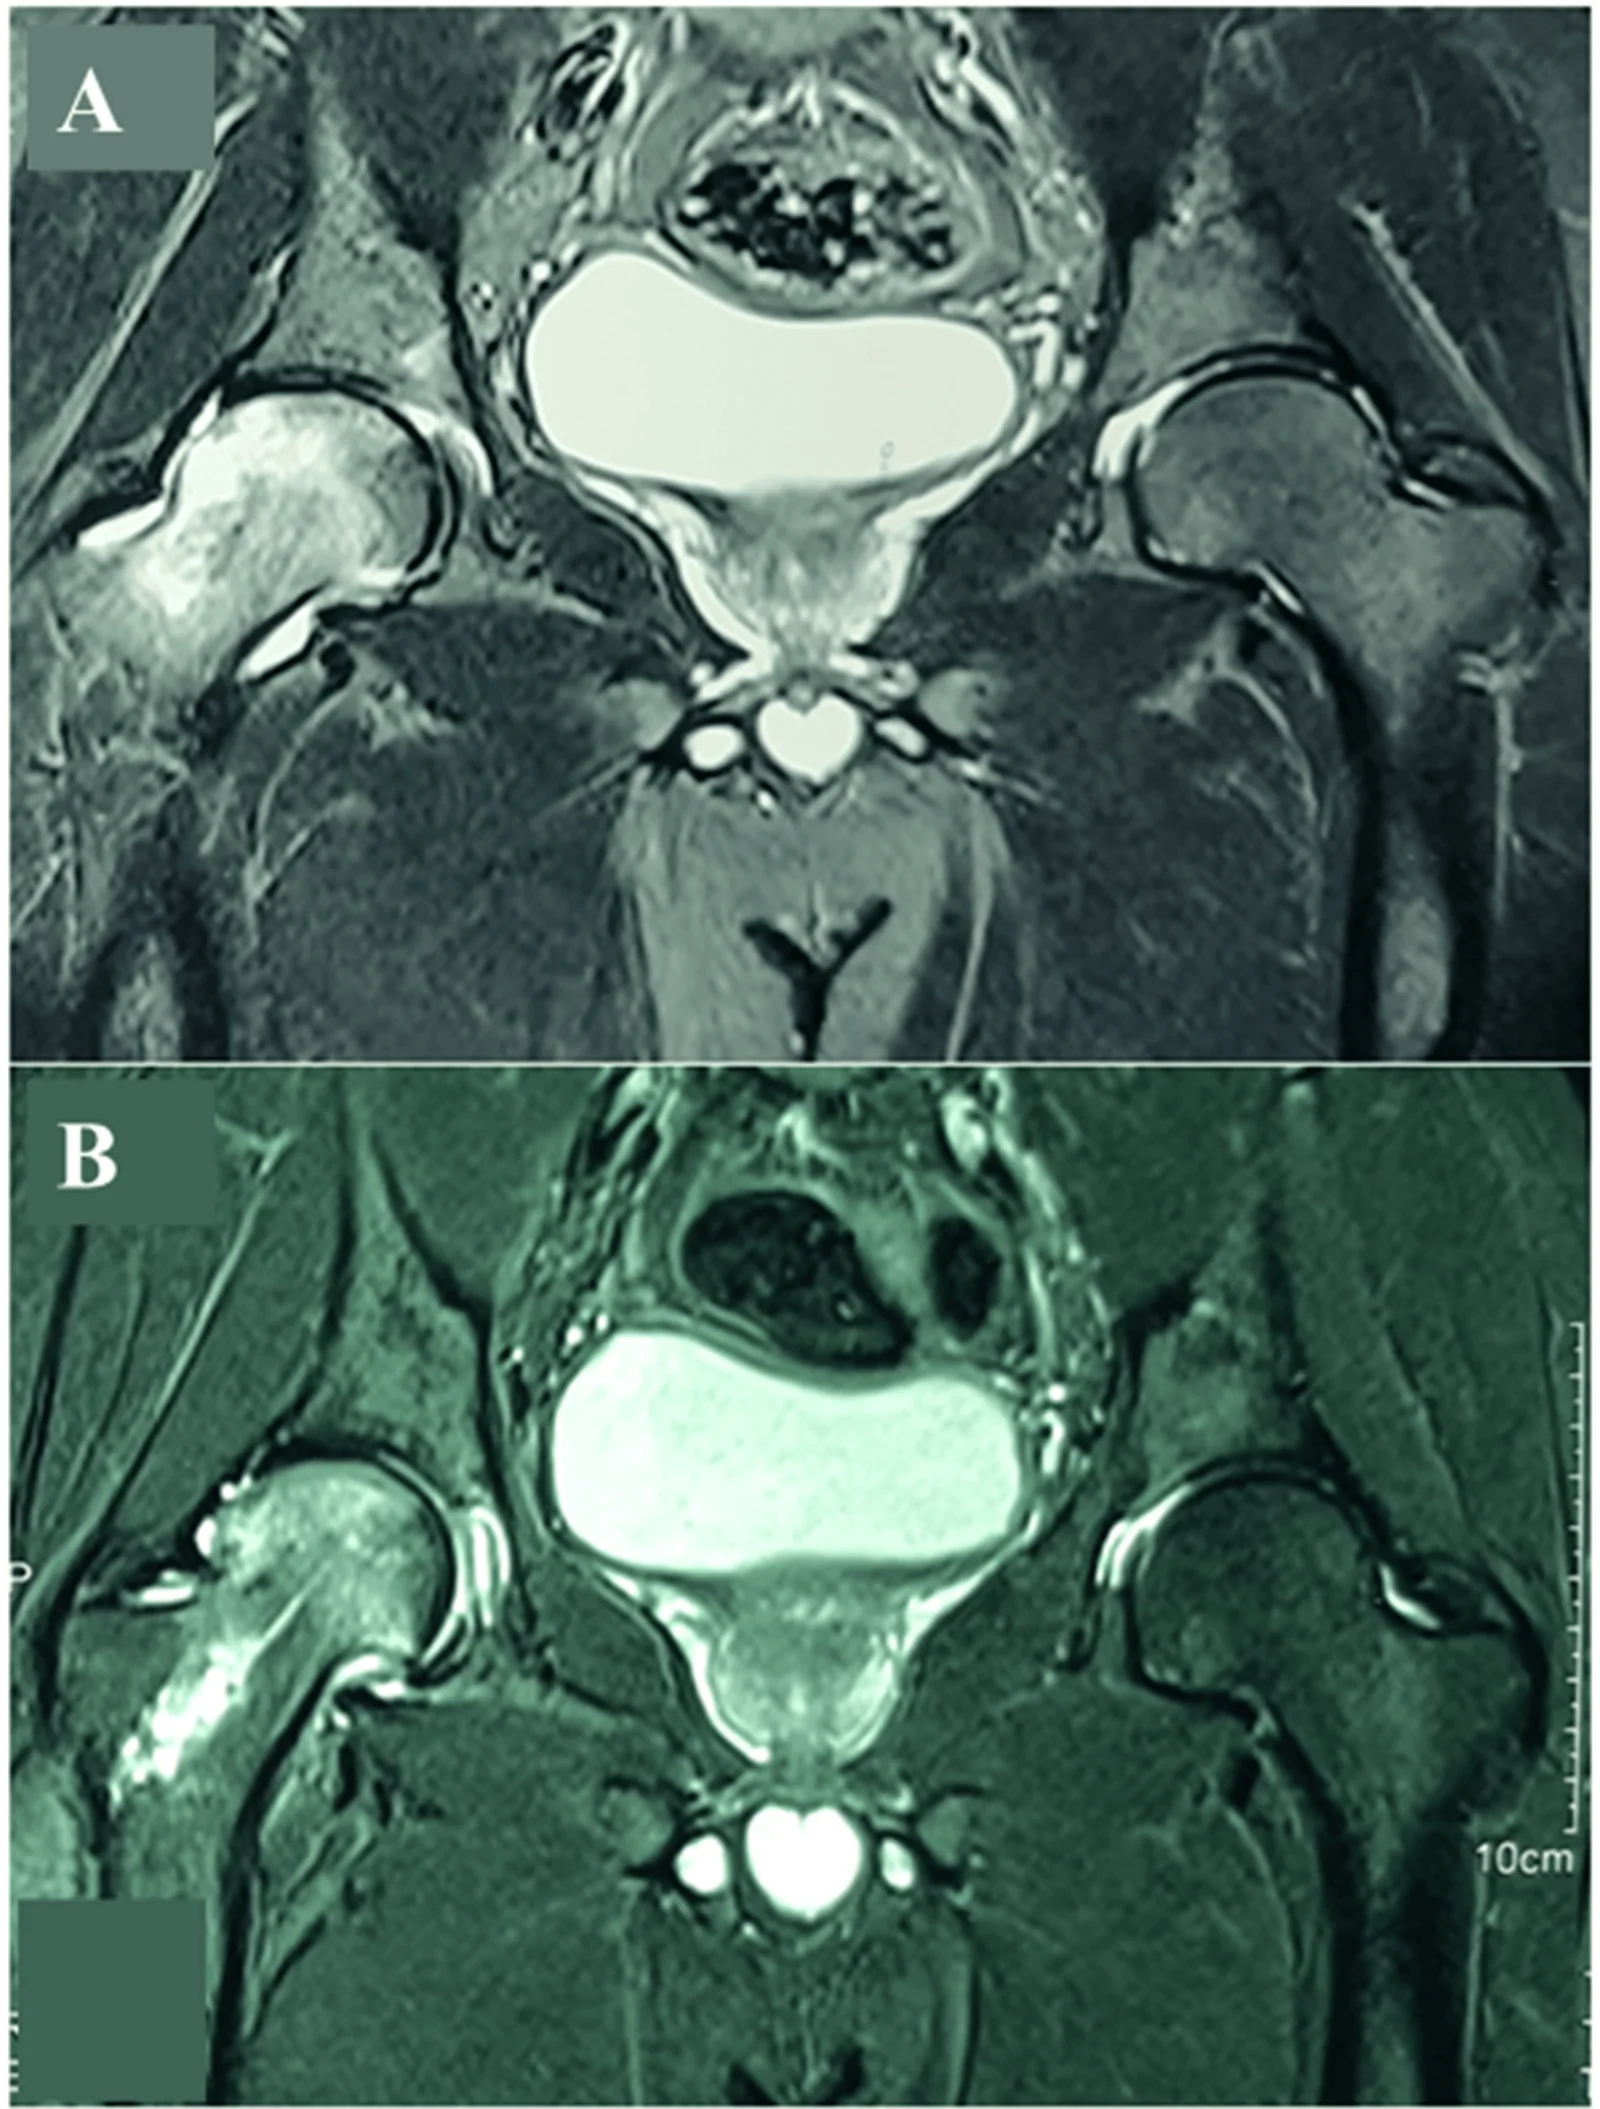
Preoperative and postoperative (36 months) MRI of hips (A) Preoperative MRI of the right hip; (B) 36-month follow-up MRI showing evidence of osteogenesis in the right femoral head, with maintenance of sphericity of right femoral head and of joint space. MRI: magnetic resonance imaging
